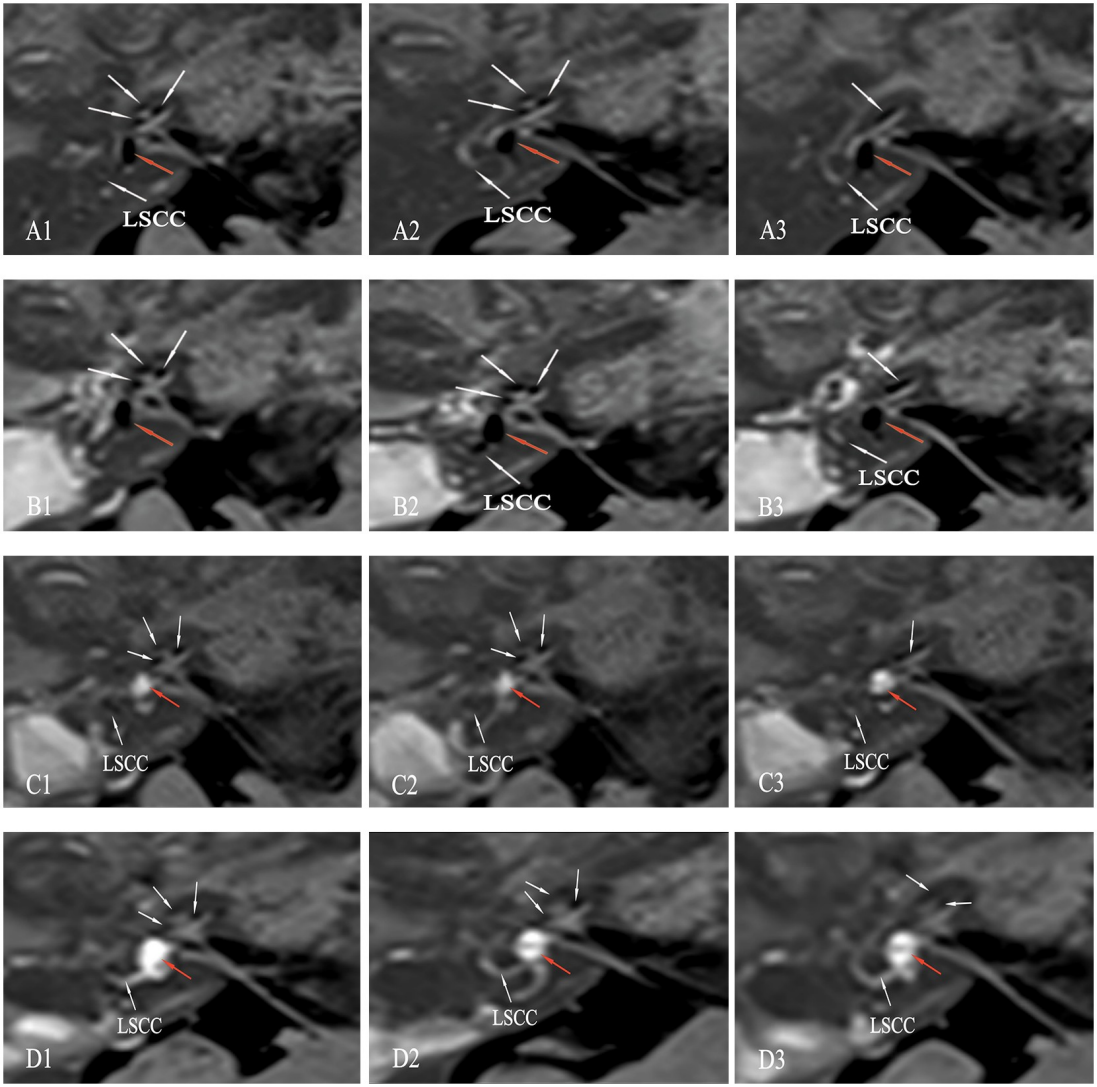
3D-real IR serial MRI scans of patient no. 14 (Table 1) with right MD. (A1-3) prior to surgery, and (B1-3) 2 weeks, (C1-3) 10 months and (D1-3) 16 months after surgery. (A1-3) Serial MRI scans showing a significant EH in the vestibule (red arrow) and cochlea (white arrow) before surgery. (B1-3) Unchanged EH in the vestibule (red arrow) and cochlea (white arrow) 2 weeks after surgery. (C1-3) Reversal of vestibular hydrops and remaining grade II EH in the cochlea 10 months after surgery. (D1-3) Serial MRI scans showing a disappearance of vestibular EH (red arrow) and the reduction of cochlear EH (white arrow) 16 months after surgery. LSCC, lateral semicircular canal; 3D-real IR, three-dimensional real inversion recovery; MRI, magnetic resonance imaging; EH, endolymphatic hydrops; MD, Meniere’s disease.
